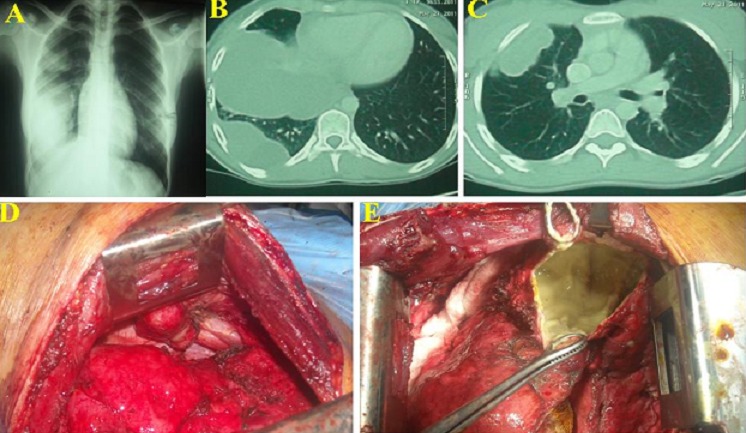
A-Chest X-ray showed multiple water opacity of the right hemithorax, B and C- Chest-CT in favour of triple location of pulmonary hydatid cyst, D- Peroperative view showed apical parietal hydatid cyst, E- Peroperative view showed large extrapulmonary hydatid cyst located between the mediastinum and diaphragm
